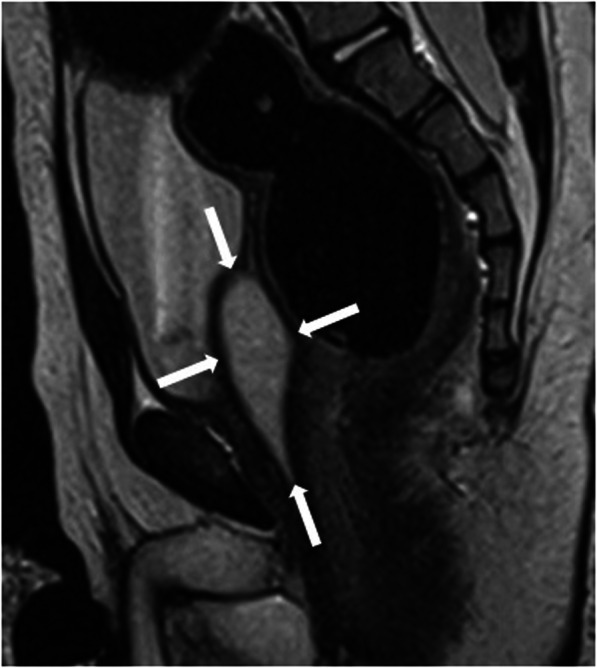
A 2-year-old male patient with recurrent urinary tract infection underwent a US exam and a midline cystic lesion was seen posterior of the bladder (not shown). Confirmatory MRI exam shows a tear-drop shaped midline cystic lesion (arrows) on the sagittal plane T2W image. The cystic lesion was extending above the posterior superior margin of the prostate, highly suggestive for the Mullerian duct cyst
